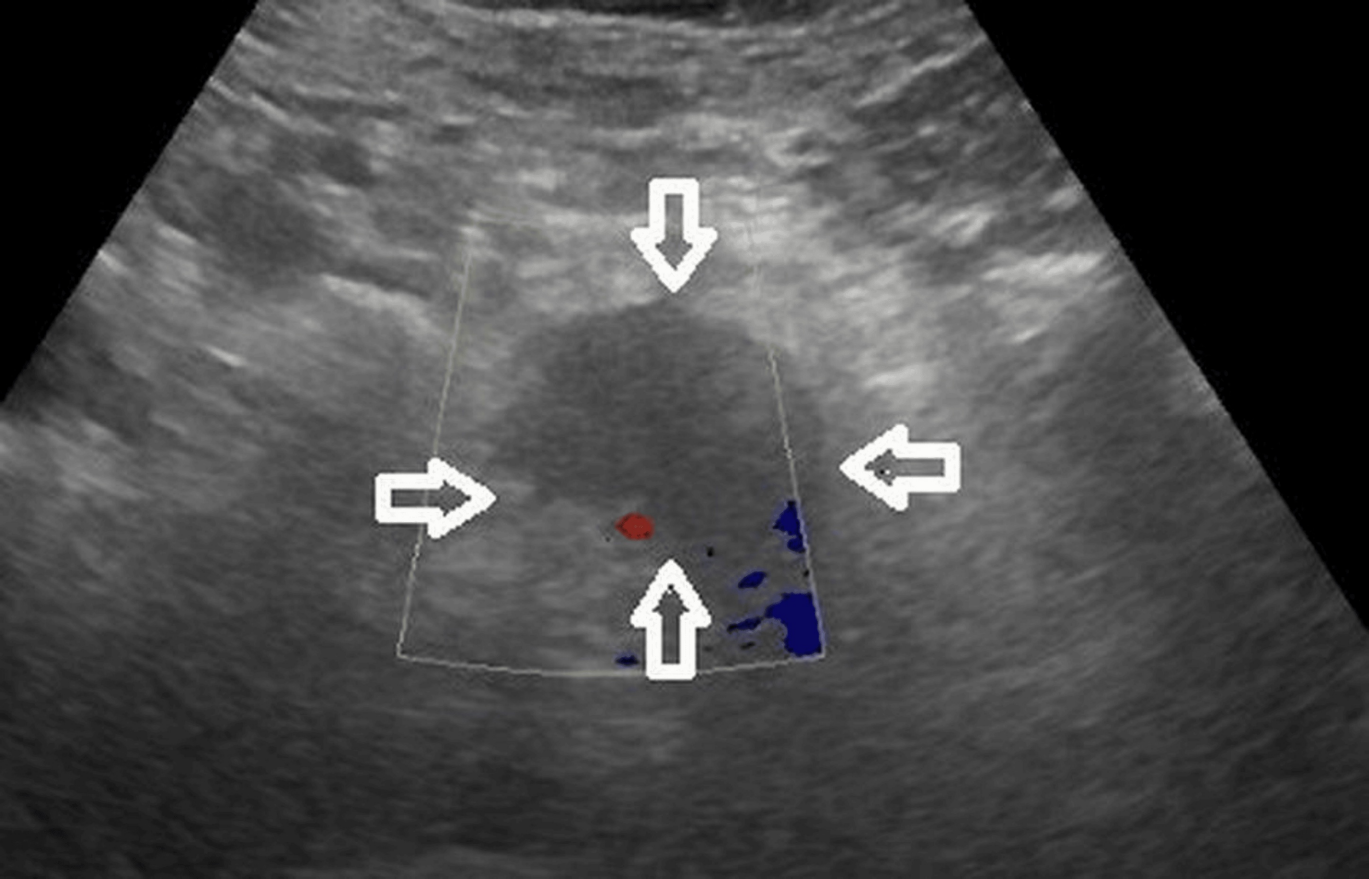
Abdominal ultrasound (US) depicting a hypoechoic pancreatic mass in the head and body of the pancreas (arrows), measuring 5.8 × 3.7 × 3.9 cm
